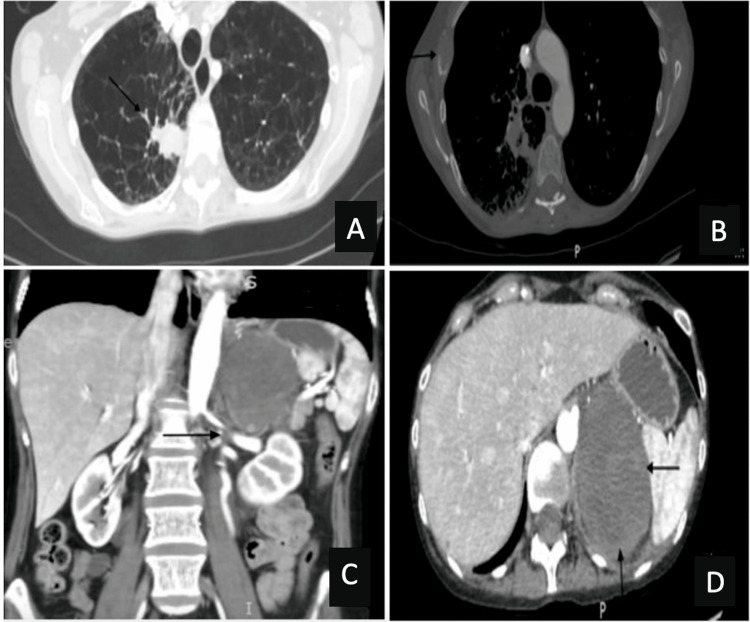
Computed tomography angiography (CTA) of the chest/abdomen and pelvis findings A. 2 cm area of consolidation in the posterior aspect of the right lower lobe in the axial plane. B. Lytic lesion involving the lateral aspect of the right fourth rib in the axial plane. C. Low-density filling defect suggestive of a thrombus in the left renal vein measuring 1.3 cm in the coronal plane. D. A large mass involving the left adrenal gland measuring 7.3 x 5.7 cm in greatest dimension which causes mass effect on the upper pole of the left kidney in the axial plane.
